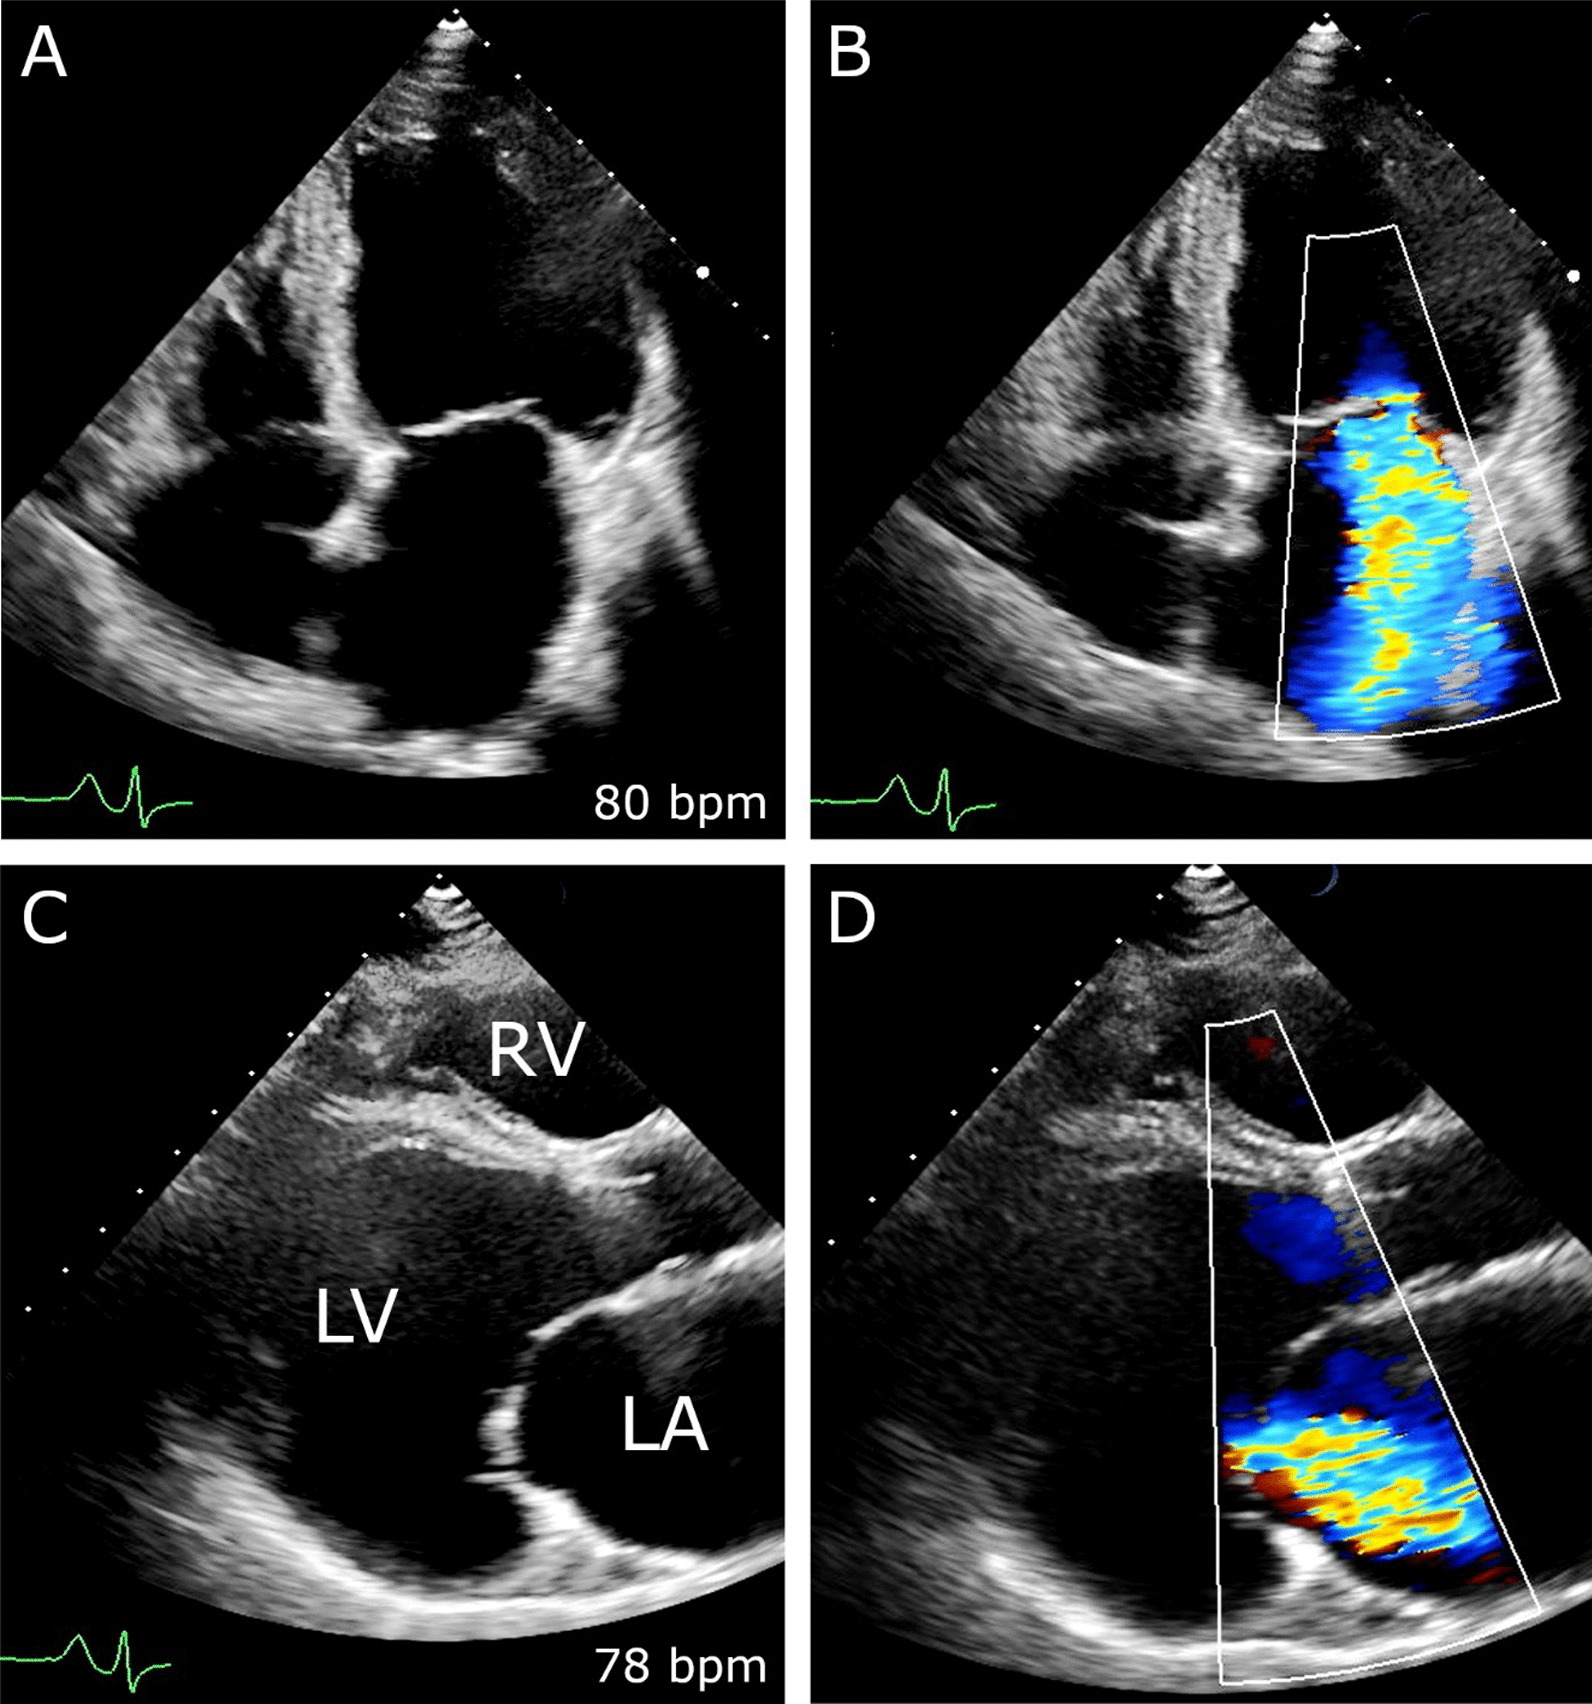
Severe FMR due to left ventricular remodeling. Preoperative transthoracic echocardiogram. Severe functional mitral regurgitation due to advanced LV distortion and mitral leaflet tethering in apical four chamber (A, B) and parasternal long-axis view (C, D)
